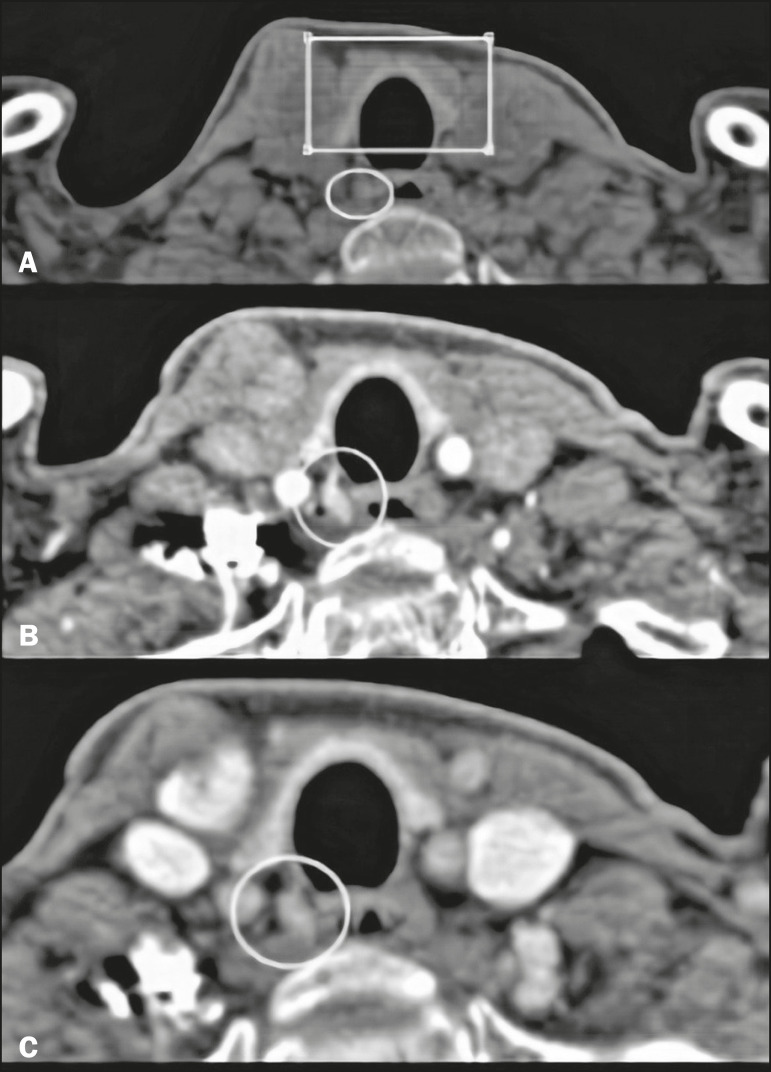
Parathyroid adenoma evaluated with the 4D CT protocol. A: Note the area of hypoattenuation (circle) adjacent to the lower third of the thyroid gland (rectangle), which is hyperattenuating in relation to adjacent structures. B: Contrast-enhanced image showing avid enhancement of the nodule (circle) in the arterial phase. C: Note the contrast wash-out in the late phase (circle).
